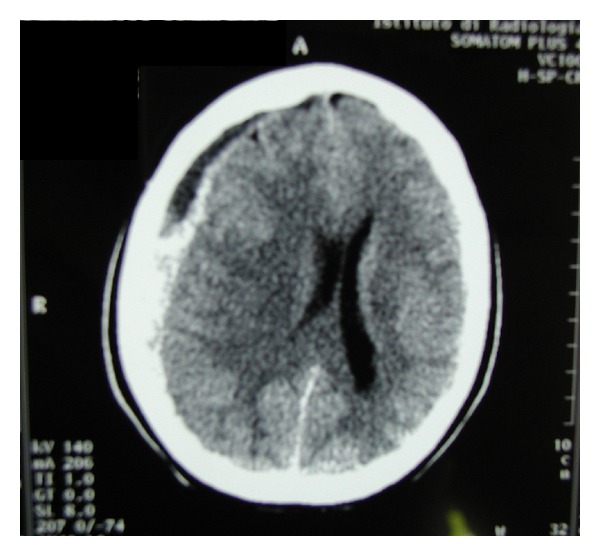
Cranial CT scan showing right sided subdural hematoma.
